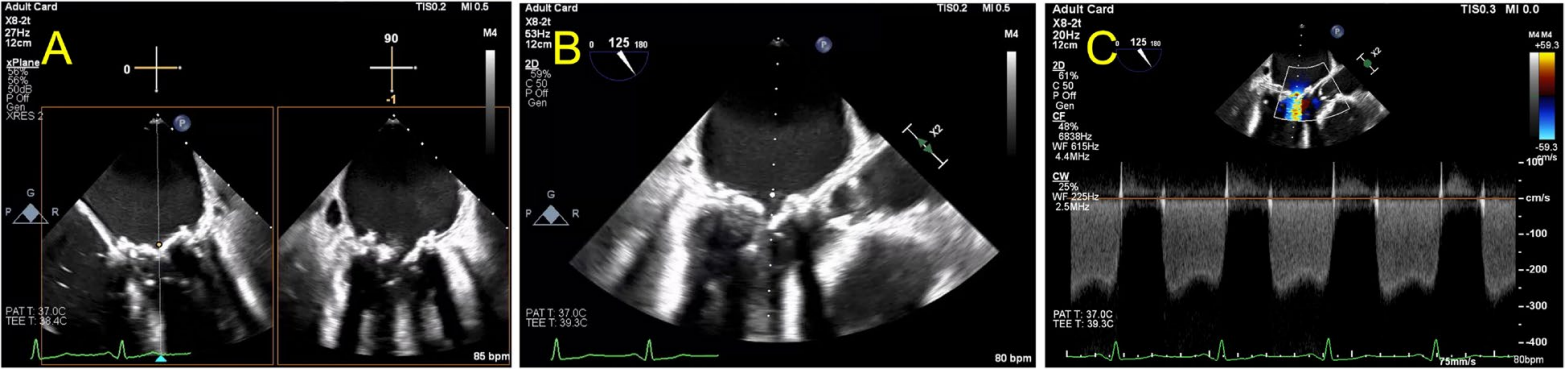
Pre-thrombus echocardiographic images of mid-esophageal 4 chamber and long axis view of bioprosthetic mitral valve. Pre-thrombus transesophageal echocardiogram (TEE) images of the bioprosthetic mitral valve in end-diastole demonstrating restricted leaflet motion in the A mid-esophageal 4 chamber view at 0 degrees and 90 degrees (biplane), B long axis view at 125 degrees and C long axis view with continuous wave Doppler envelope consistent with severe mitral stenosis with peak velocity of 2.5 m/sec
